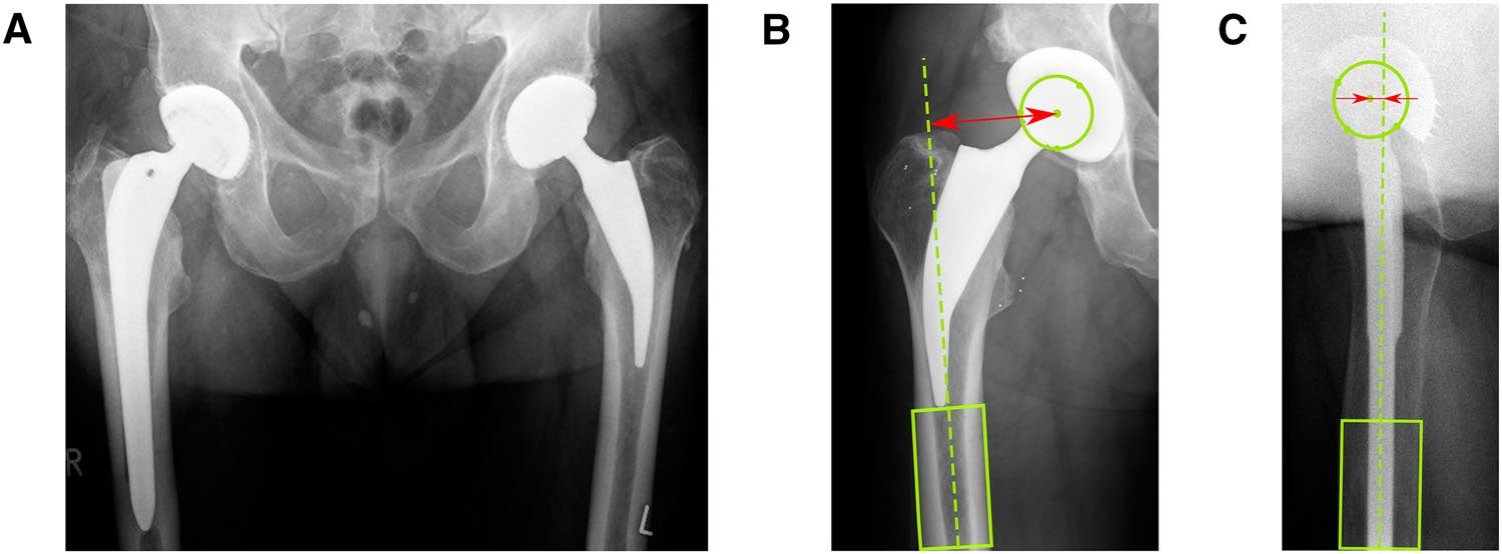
(A) Pelvic x-ray of a patient with a conventional hip stem (Bicontact) on the right side and a short hip stem (Metha) on the left side. (B) Measurement of the lateral offset on a clinical x-ray. (C) Measurement of the anterior offset in a Johansson positioning.
